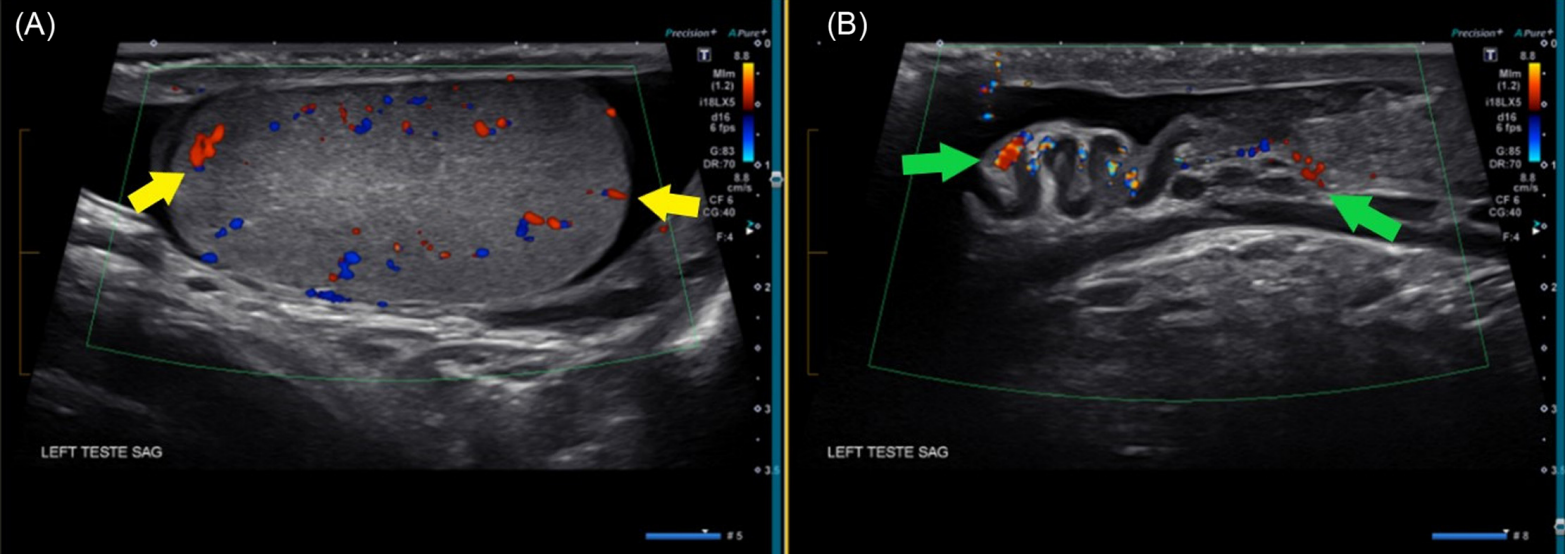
Repeat Doppler ultrasound of the left testicle after manual detorsion. (A) Sagittal view of the left testicle showing restored arterial and venous flow (yellow arrows). (B) Sagittal view of the spermatic cord and epididymis with resolution of the heterogenous mass and restoration of blood flow (green arrows), consistent with successful detorsion.
